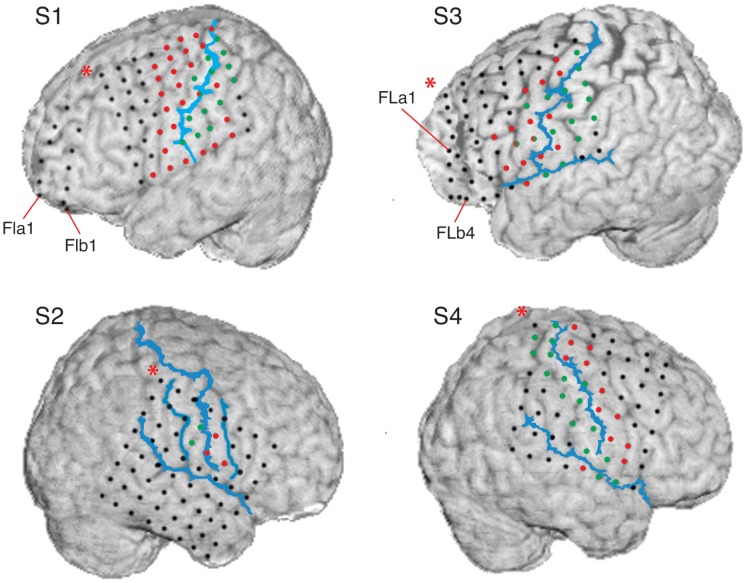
Locations of ECoG grid electrodes in relation to the cortex. Electrode positions (red, green and black circles) were reconstructed from the post-implantation MRI scan and positioned over the pre-implantation MRI scan [88]. For S1, S3 and S4 red (green) circles represent electrodes that showed motor (somatosensory) responses to electrical stimulation mapping (ESM). For S2, motor and somatosensory electrodes were determined from sulci reconstruction. Central sulci, Sylvian fissures and, for S2 only, pre and post central sulcus are shown as blue lines. These were drawn by hand to resemble sulci reconstruction from the post-implantation MRI scan. Each of the subjects was implanted with an 8×8 ECoG grid. In S2, no recordings were made from the top row of the ECoG grid. In addition to ECoG grids, the Figure shows two 6 electrode ECoG strips over the frontal lobe (FLa and FLb) for S1 and two 4 electrode ECoG strips (FBa and FBb) over the frontal lobe for S3. In this study, we analyzed the recording from the ECoG grids only.
